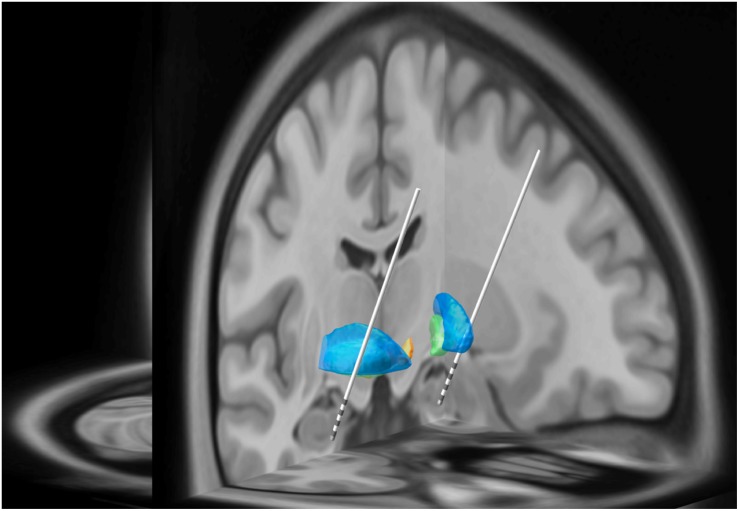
In this three-dimensional image, Subject 2’s MRI brain imaging studies were registered to the MNI space (MNI152 NLIN2009a) and then overlaid on the structural atlas of Mai (Horn and Kühn, 2015; Mai and Majtanik, 2017). Here we demonstrate the trajectory for each DBS electrode implanted into the head of the HC and amygdala as represented in “MNI space.” The blue regions represent the putamen while the green regions represent the globus pallidus. The white lines represent the DBS leads with four gray regions representing individual electrode contacts. See Figure 2 for precise localization of each contact.
